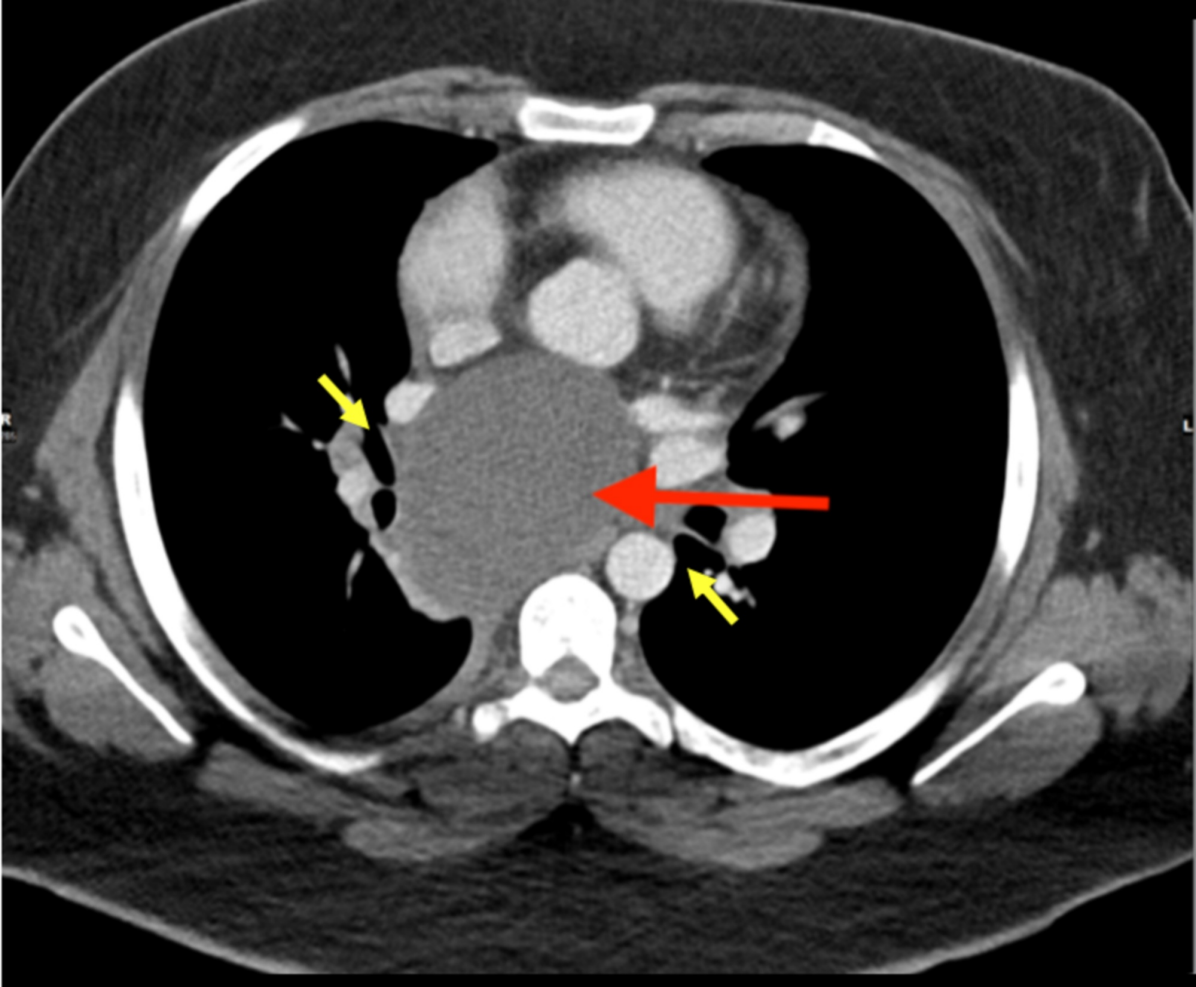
Chest CT (axial view) with intravenous contrast showing a 7.5 x 6.8 x 6.3 cm mildly complex fluid-filled thin-walled subcarinal mass (red arrow). The mass is splaying the right and left mainstem bronchi (yellow arrows).
